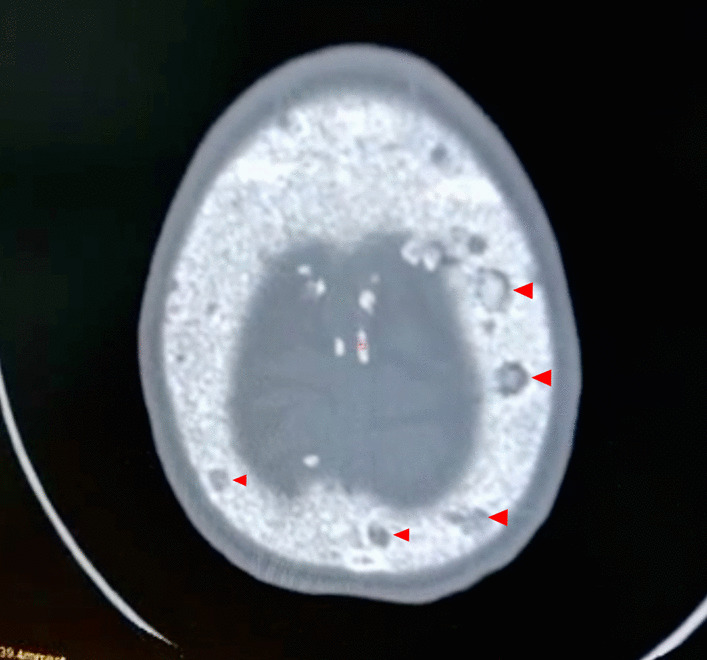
Skull CT. Lytic lesions in the skull bone (arrowheads)
